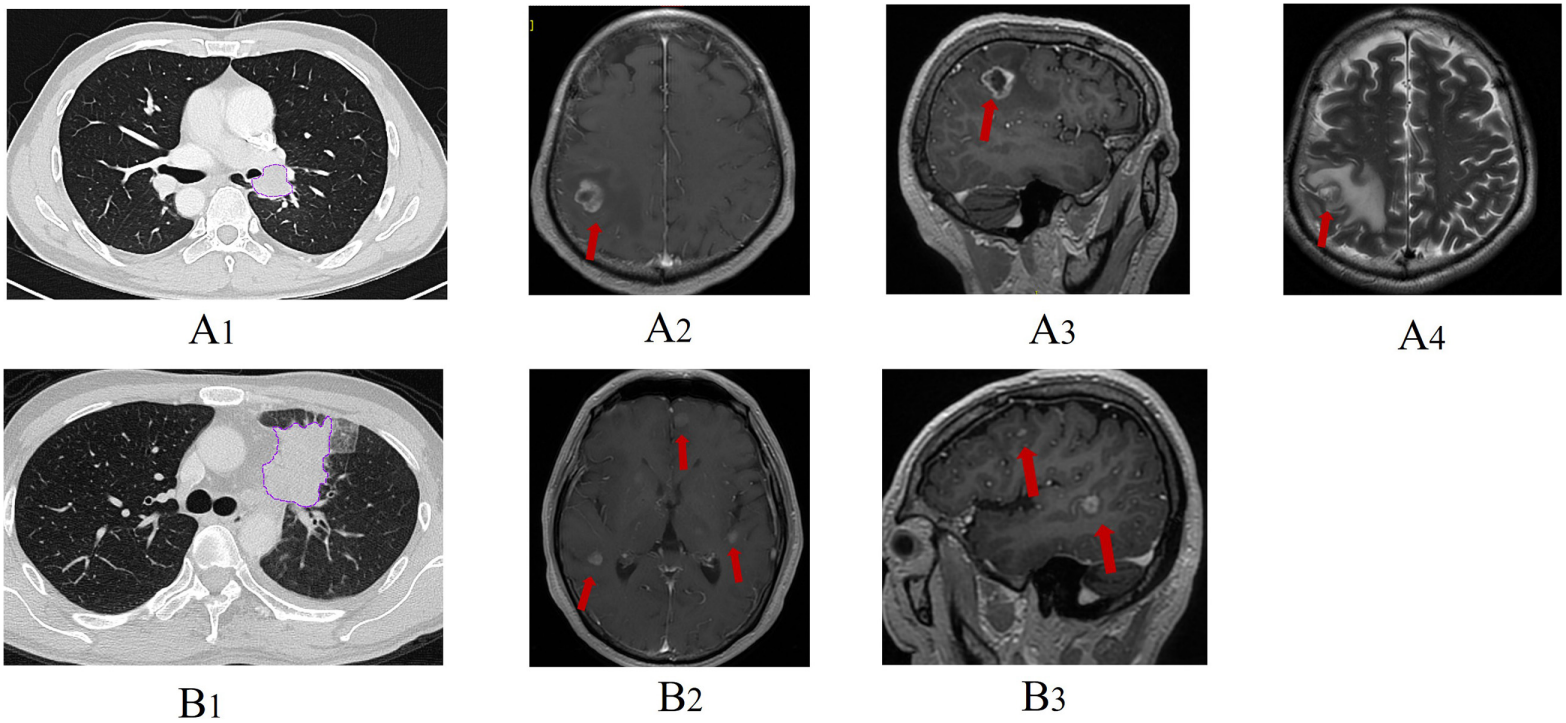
Representative clinical cases and related thoracic CT/brain MRI images. One patient had a pre-treatment thoracic CT image (A1) and brain MRI image (A2-A4) which indicated brain metastasis at baseline examination. Another patient (B1) was free from brain metastasis at baseline evaluation but developed brain metastasis after chemotherapy (B2, B3, arrow).
